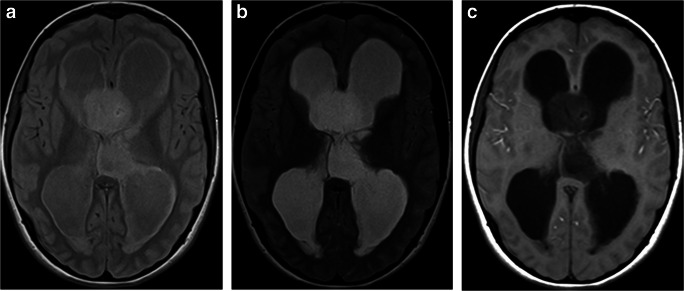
Septal dysembryoplastic neuroepithelial tumor. a An axial T2 FLAIR image through the level of the foramen of Monro demonstrates a minimally hyperintense mass causing obstructive hydrocephalus. b The mass is barely visible on the T2-weighted sequence because the signal intensity of the mass is similar to that of ventricular CSF. c The post-contrast T1-weighted sequence demonstrates no enhancement of the mass
